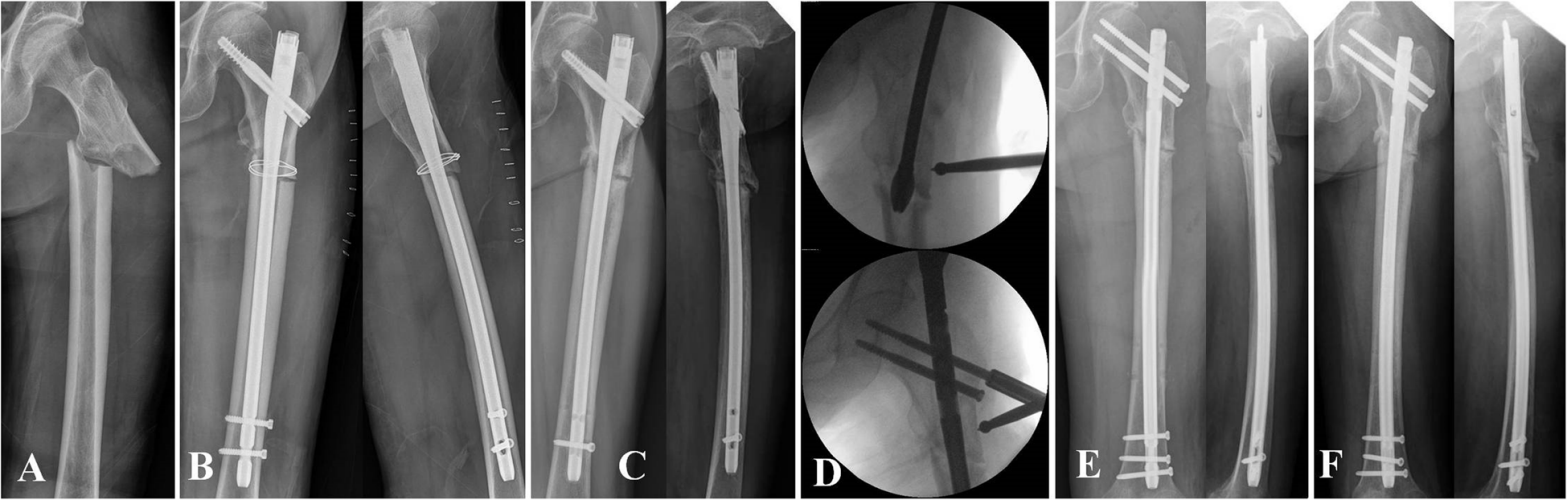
a A 68-year-old female patient who sustained atypical subtrochanteric fracture by low-energy injury. b Postoperative radiographs after open surgery. She underwent a total of three open surgeries. c Nonunion and implant failure at 43 months after initial surgery. d Revision surgery using closed procedure without bone graft. e Postoperative radiograph. f Nonunion at 1 year after revision surgery
